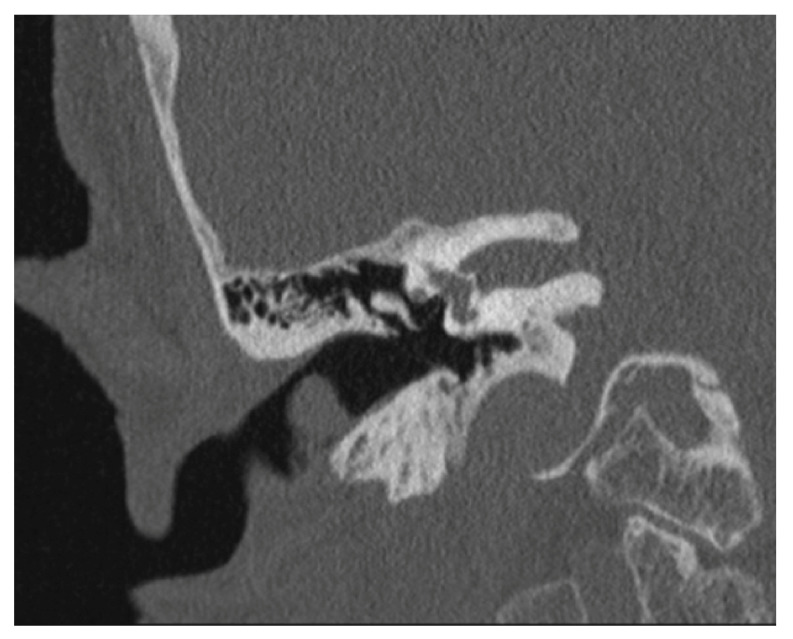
Computed tomography imaging (coronal plane) shows a homogeneous tumor in the right external auditory canal without evidence of bone erosion.
